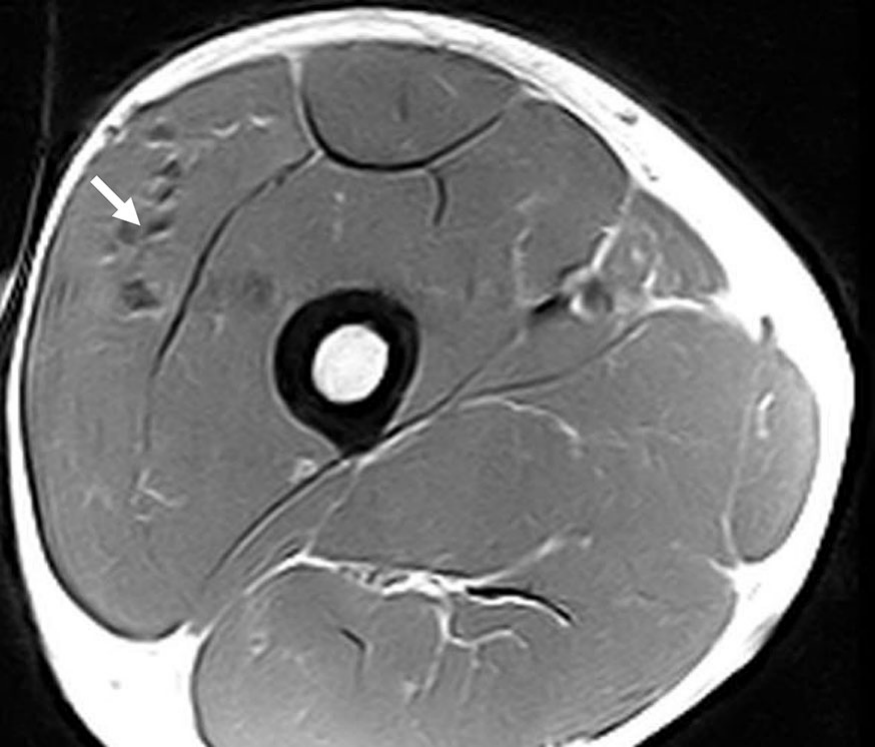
MRI image from a young man showing connective tissue accumulations around the vastus lateralis motor point (*arrow*). The surface-recorded CMAP was 66 % smaller, the sMUP was 60 % smaller and the MUPs were 46 % larger than average for young men
